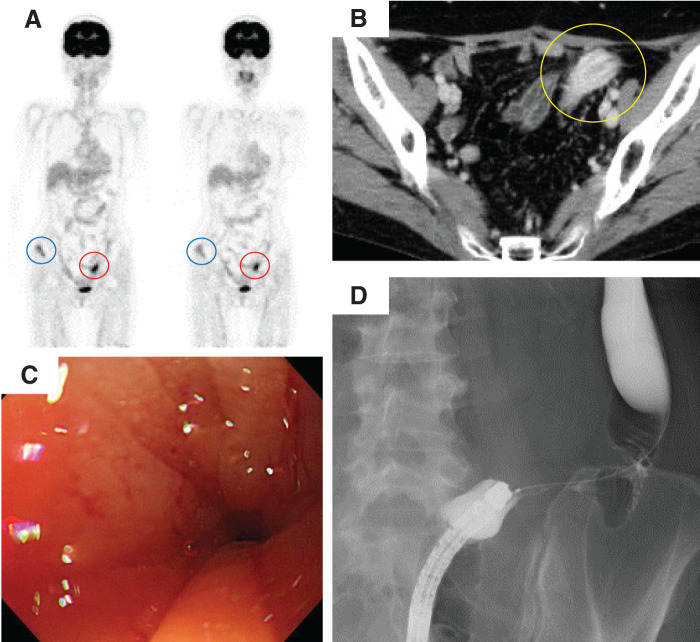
Imaging and endoscopic findings of sigmoid colon lesion. (A) PET-CT images showing FDG uptake in the right iliac bone (blue circles), as previously noted, as well as new accumulation in the sigmoid colon (red circles). (B) Contrast-enhanced CT revealed a mass lesion with enhancement in the sigmoid colon (yellow circle). (C) Colonoscopy demonstrated severe luminal narrowing in the sigmoid colon, preventing scope passage. No overt mucosal tumor was observed. (D) Contrast enema performed concurrently with colonoscopy revealed a tight stricture in the sigmoid colon, although a guidewire was able to pass through.
